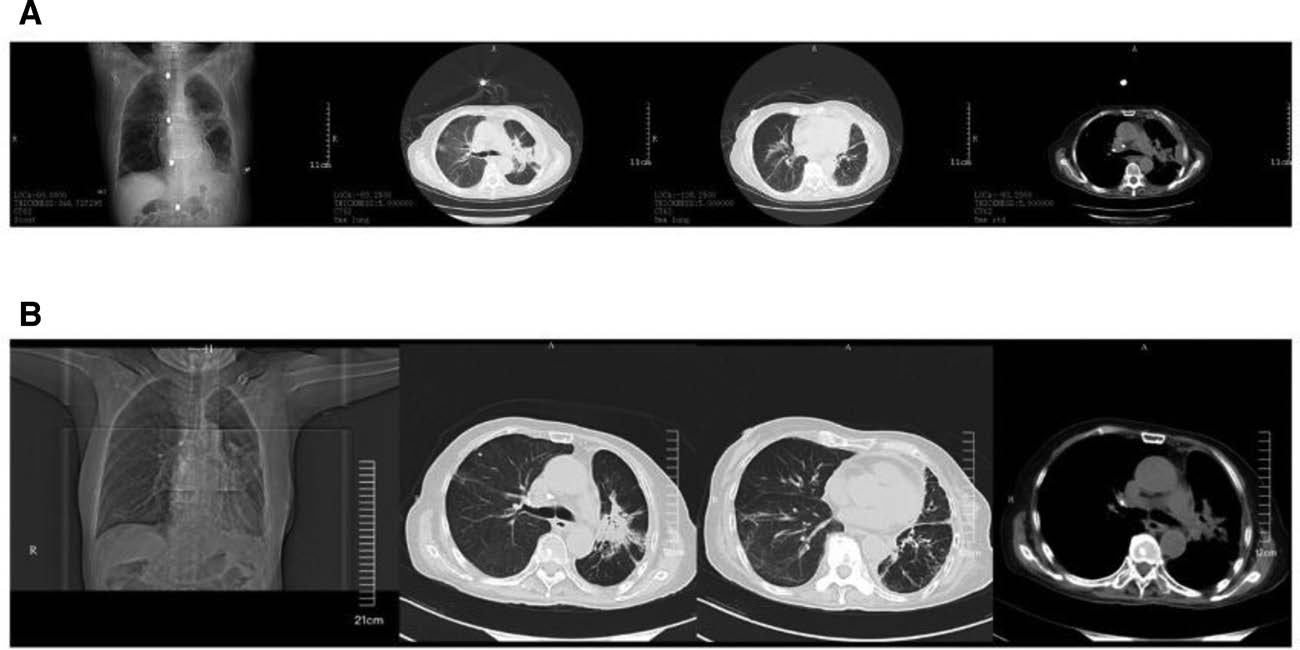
Follow-up chest CT after discharge (A) Chest CT was reviewed in March 2022 (B) Chest CT was reviewed in July 2022. CT = computed tomography.
